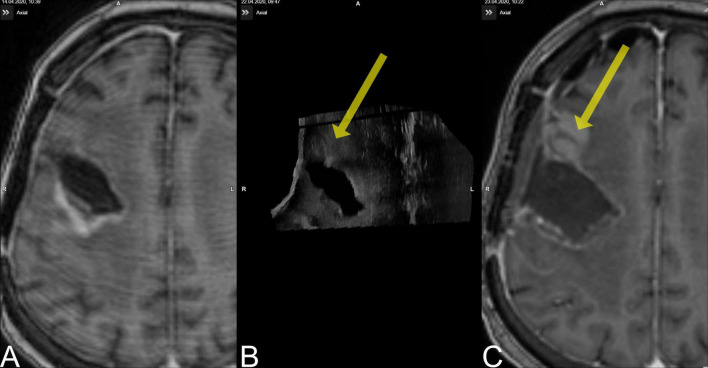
Case no. 9. (A) The pre-MRI (8 days prior to surgery) demonstrated a contrast-enhancing lesion occipital to the resection cavity. (B) The pre-US demonstrated a lesion rostral to the resection cavity (yellow arrow) that was missed during surgery. (C) The new lesion is evident on post-MRI.
